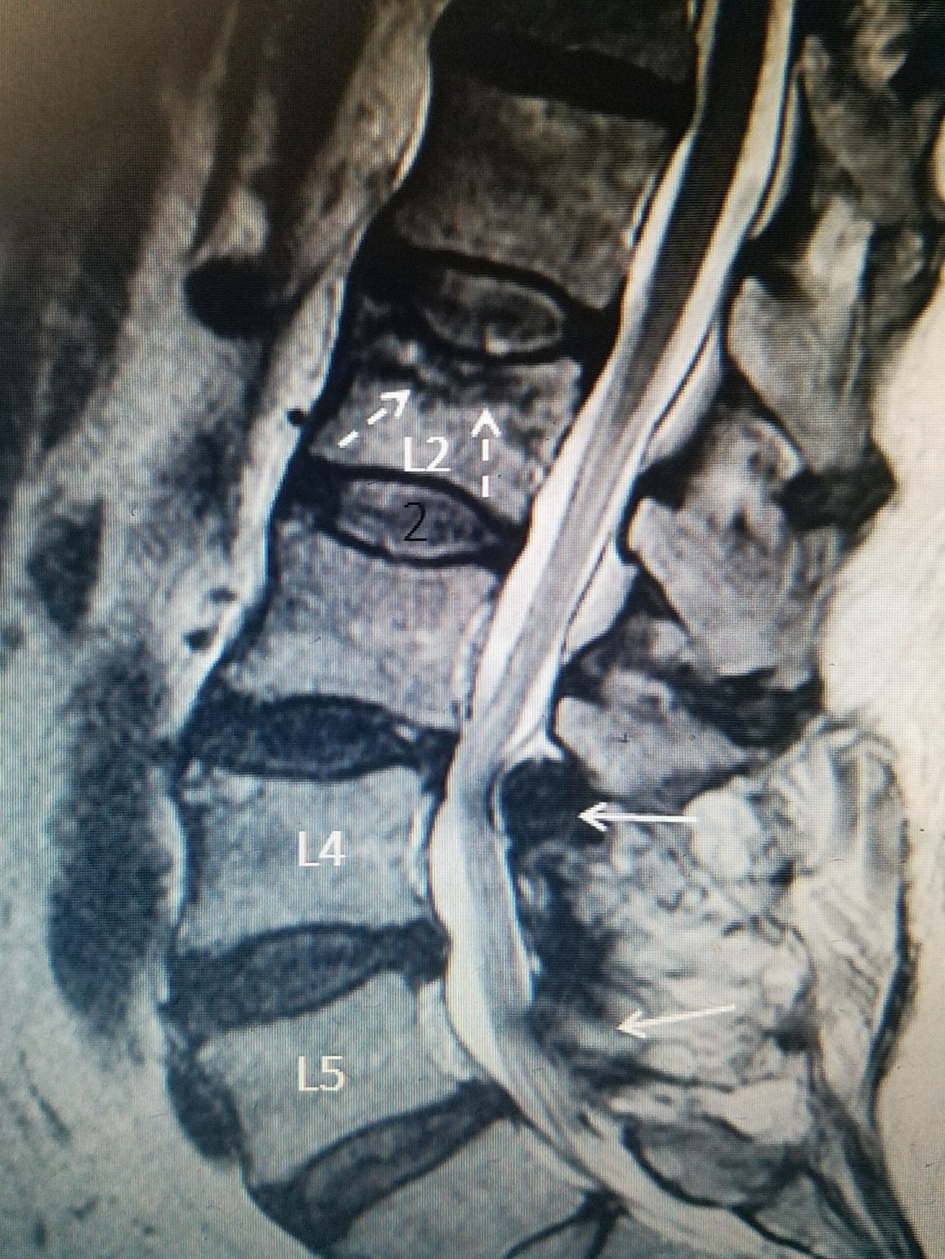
L2 fracture 15 years after lumbar fusion above post fusion stenosis after previous L4 to S1 fusion Sagittal T2 magnetic resonance imaging (MRI) scan: Patient is a 68-year-old female with previous L4-S1 laminectomy and posterior fusion 15 years previously without instrumentation. There is development of post fusion stenosis at L3-4 (solid white arrows). After a fall, she developed mid lumbar pain. MRI revealed an acute superior endplate compression fracture at L2 (dotted white arrows).
